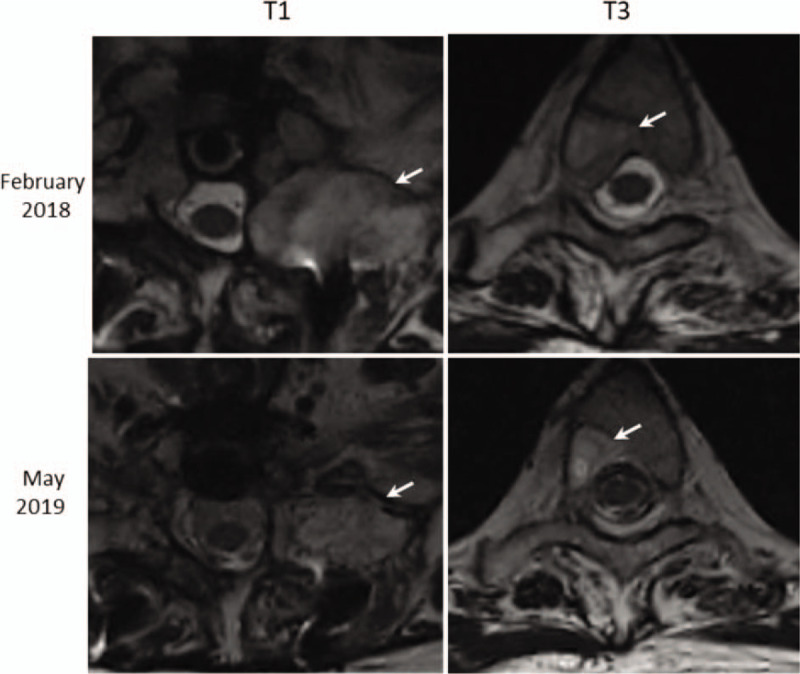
Magnetic resonance imaging of the breast cancer patient with relapse of bone metastases in February, 2018 and on follow-up in May 2019. The tumor on the left side of the vertebral T1 has shrunk from 35.2 to 28.1 mm. Another one on the right side of the T3 vertebrae has shrunk from 12.0 to 9.9 mm. The arrow indicates the tumor.
